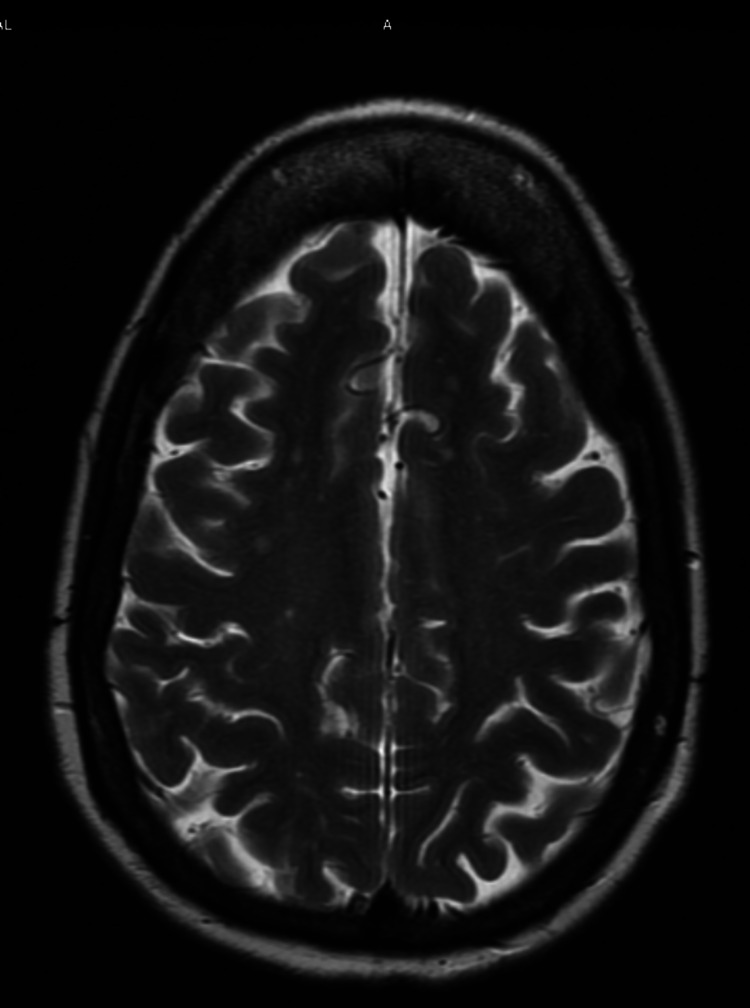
MRI brain showing microangiopathic changes
